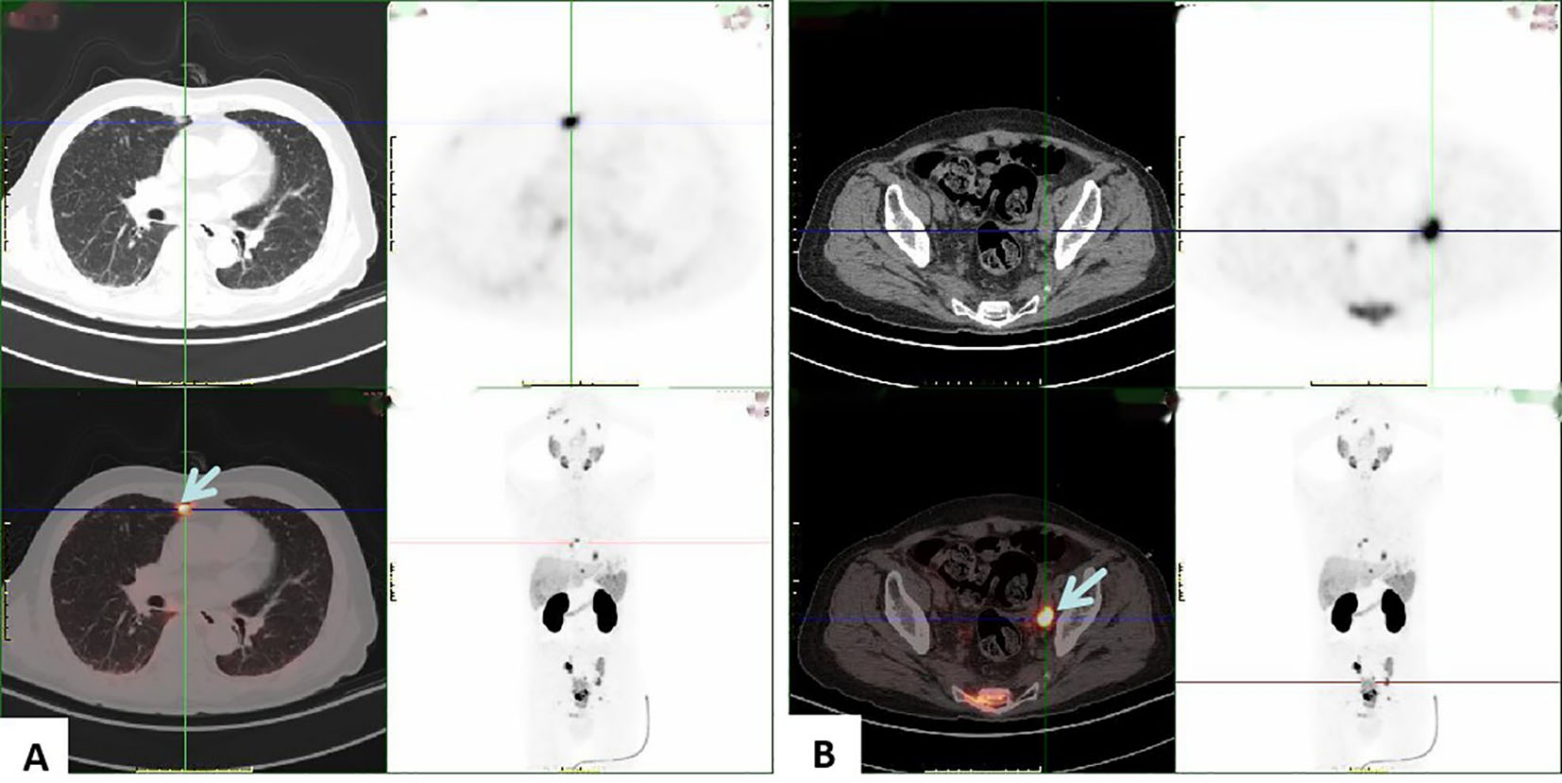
PSMA PET/CT shows high uptake in the lungs (A) and left iliac vascular lymph nodes (B), suggesting metastasis to the lungs and left iliac vascular lymph nodes from prostatic cancer.
